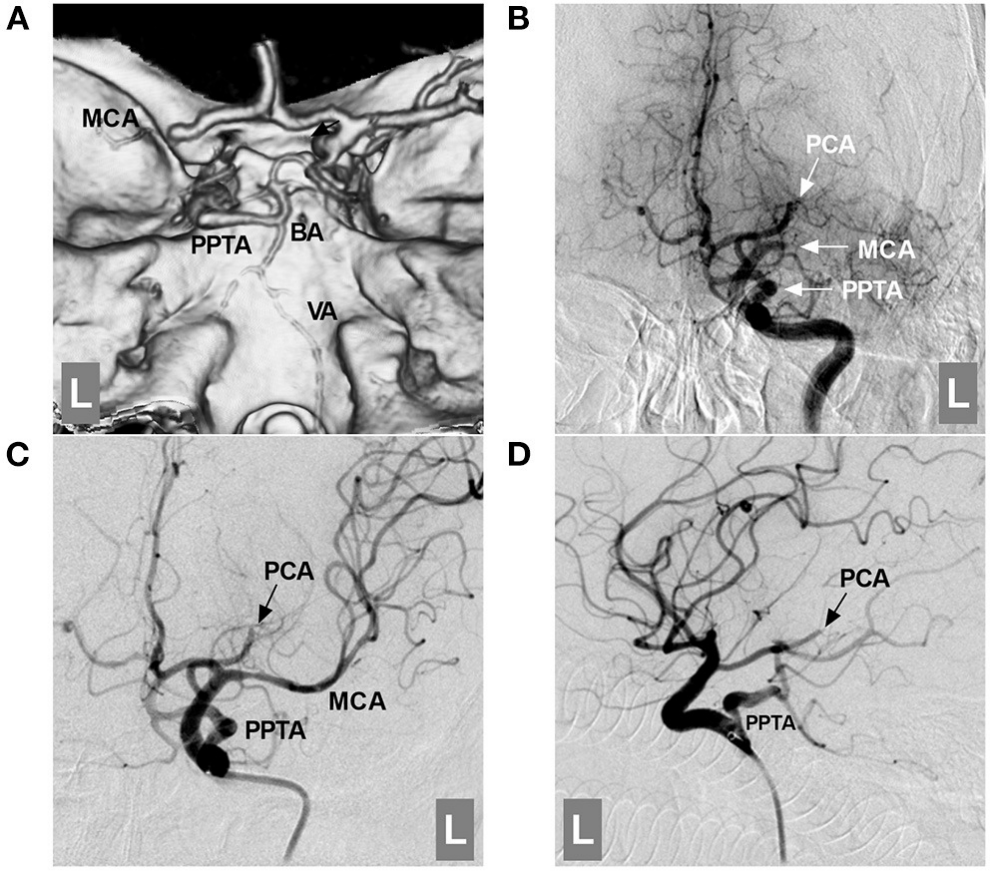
Role of the PPTA in acute large vessel occlusion. (A): CTA showing an occluded left MCA and a PPTA connected with the BA; the proximal BA and VA were hypoplastic. (B): DSA of the ICA showing an occluded left MCA and PCA (arrows with MCA and PCA); the PPTA (arrow with PPTA) is unaffected. (C,D): DSA of the ICA showing that the MCA was revascularized after mechanical thrombectomy; the PCA was fetal-type and occluded distally (arrows with PCA). BA, basilar artery; CTA, computed tomography angiography; DSA, digital subtraction angiography; ICA, internal carotid artery; L, left; MCA, middle cerebral artery; PCA, posterior cerebral artery; PPTA, persistent primitive trigeminal artery; VA, vertebral artery.
